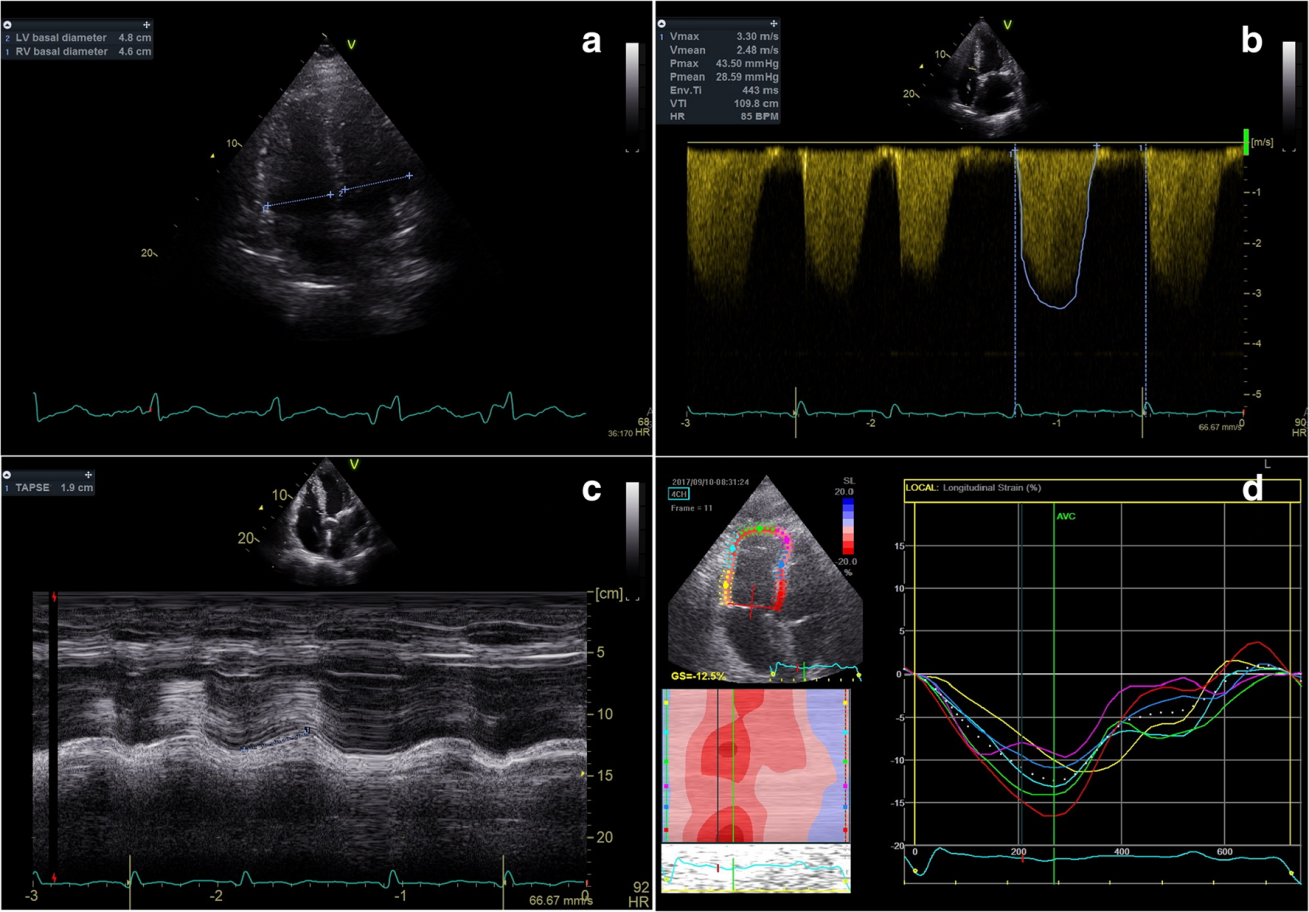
Relevant echocardiographic parameters found in acute pulmonary embolism. This figure shows the most common findings that can be found in the setting of acute pulmonary embolism. (a) Ratio between right ventricular (RV) and left ventricular (LV) basal diameters, often enough > 1, meaning a RV dilatation. (b) Right atrio-ventricular gradient, rarely above 60 mm Hg in the acute setting. (c) Tricuspid annular plane systolic excursion (TAPSE), which in acute setting is not necessarily reduced compared with controls.(d) Reduced RV longitudinal strain
